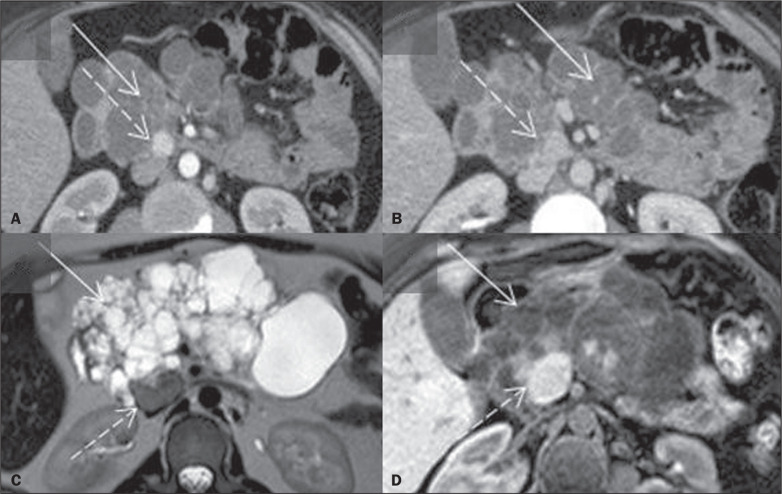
Contrast-enhanced axial CT scan, in the arterial and nephrographic phases
